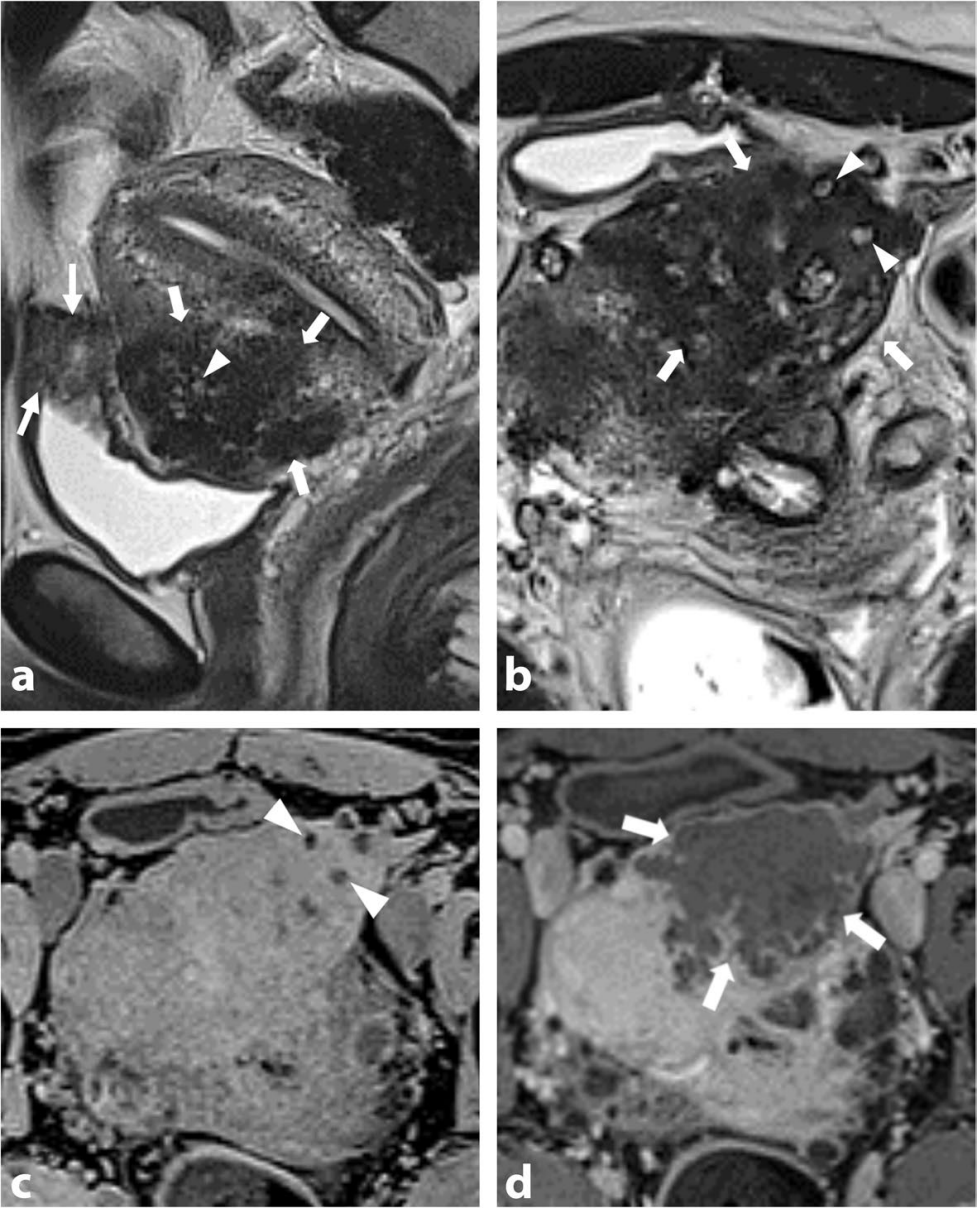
Pelvic alveolar echinococcosis in a 28-year-old woman with chronic pelvic pain, bladder disorder, and dyspareunia. a Sagittal and (b) axial T2-W MR images show an infiltrative T2-hypointense external myometrial infiltrative mass (thick arrows) with a few microcysts (arrowheads), and a contiguous infiltration of the bladder dome (thin arrows). c Axial T1-W fat-suppressed MR image shows several microcysts (arrowheads) without hemorrhagic foci in the extrinsic infiltrative uterine mass. d Axial T1-W fat-suppressed contrast-enhanced subtracted MR image shows central necrosis (arrows) and irregular margin with a peripheral enhancement. History of liver alveolar echinococcosis infection in childhood was then found
